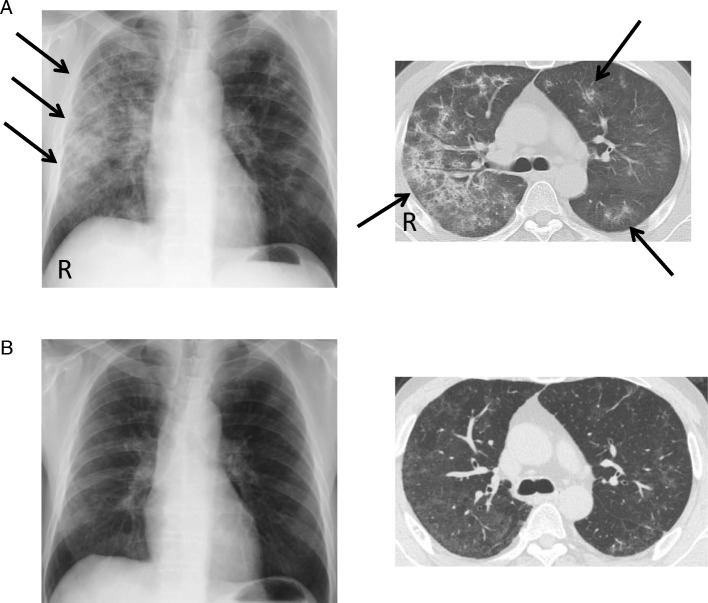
(a) Chest X-ray and CT radiography of the 61-year-old man of IP show consolidation in bilateral lungs, dominantly in the lower and right side (arrows). (b) On the 24th day after treatment with oral PSL, the consolidations are improved. R: right
